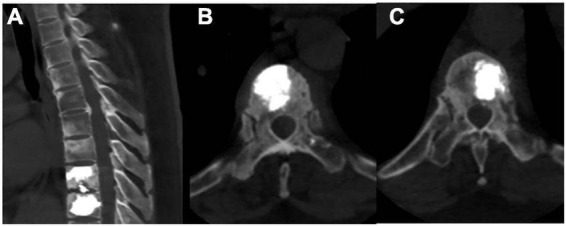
Post-operative radiographic images (A–C). (A–C) Post-operative thoracic CT revealed that the bone cement in T8 and T9 vertebrae was well dispersed.
